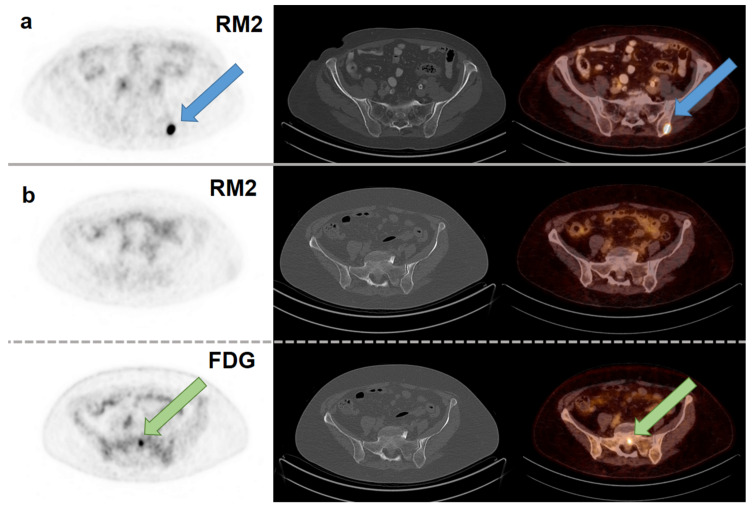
Axial slices of PET scans (first column), CT scans (second column) and fusion images (third column). (a) [^68^Ga]RM2 PET/CT of a 73-year-old patient (patient no. 3) with a bone metastasis in the left iliac bone with intense RM2 binding and not seen on CT (blue arrow; SUVmax 32.1; first row). (b) [^68^Ga]RM2 PET/CT of a 75-year-old patient (patient no. 5) with a bone metastasis in the sacrum without RM2 binding and not seen on CT (SUVmax 1.5; second row). [^18^F]FDG PET/CT of the same patient with intense hypermetabolism in the sacrum (green arrow; SUVmax 5.8; third row). Note the physiologically higher tracer uptake in healthy bone on [^18^F]FDG PET. Fixed inversed grey-scale images are displayed with an SUV window of 0–5.
